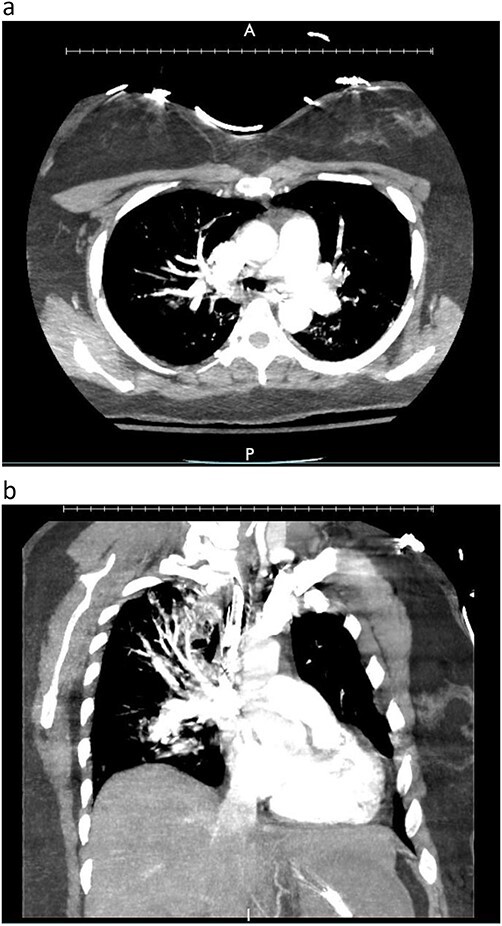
Donor angio CT scan: (a) filling in the left PA, (b) reduction of blood supply of the left lung.
